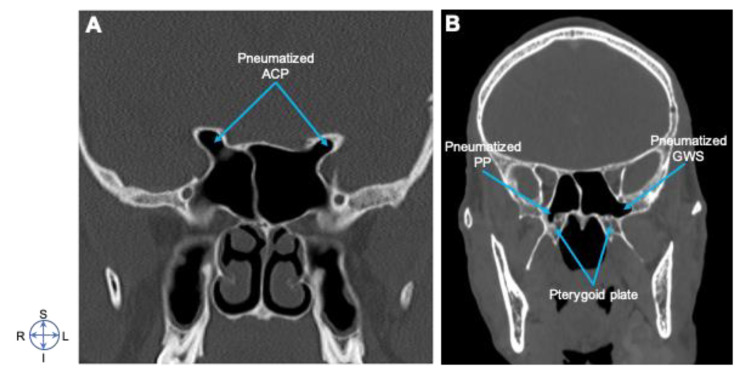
Pneumatization of the sphenoid sinus. Coronal CT images showing (A) Pneumatized anterior clinoid process (ACP); (B) Pneumatized pterygoid plate (PP) and greater wing of sphenoid (GWS). Note that half of the patient population participating in the study possessed sellar type of sphenoid sinus pneumatization. Image orientation: S, superior; I, inferior; R, right side; L, left side.
